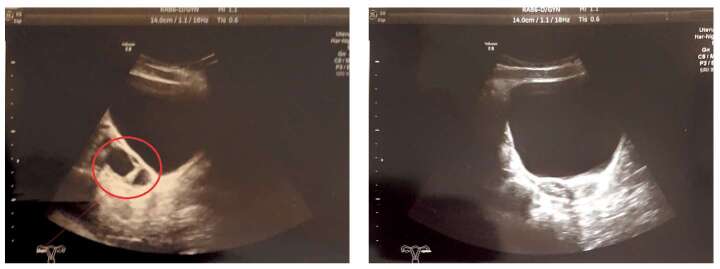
Рисунок 3. УЗИ органов малого таза, выполненное в 9,2 года. В правом яичнике визуализируются две кисты; левый яичник без особенностей.Figure 3. Ultrasound of the pelvic organs, performed at 9.2 years. Two cysts are visualized in the right ovary; the left ovary is unremarkable.
